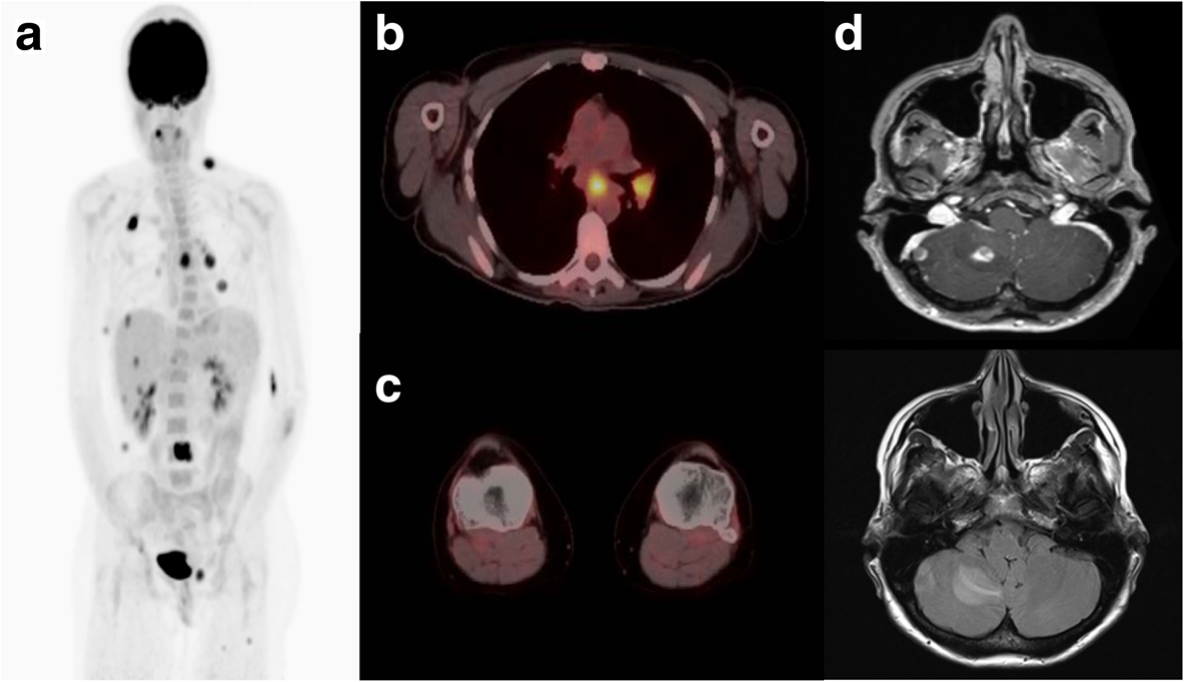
Imaging at Time of Diagnosis Before Treatment. a Scout PET scan showing cervical/mediastinal/inguinal adenopathy and vertebral lesions. b Cross section of mediastinum with increased update in mediastinal nodes (SUV_max_ 10 in subcarinal node). c Cross section of knees with no increased uptake. d MRI brain with two right cerebellar metastases with vasogenic edema
